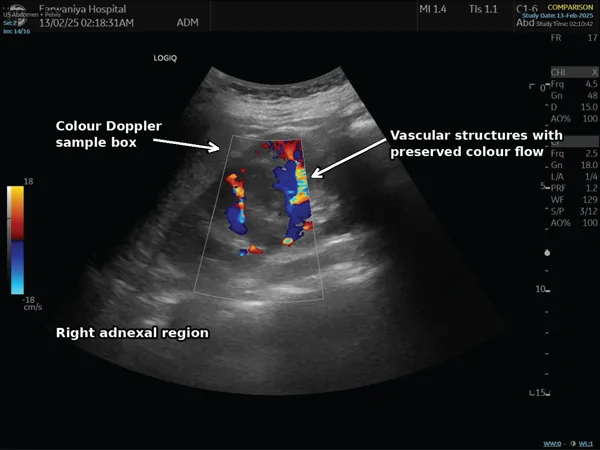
Transabdominal colour Doppler ultrasound of the right adnexal region. The colour Doppler sample box and vascular structures showing preserved colour flow are annotated. The small simple right adnexal cystic lesion of approximately 2.5–3 cm described in the contemporaneous radiology report is not resolved on this archived static image; the full sonographic findings are reported in the text. Preserved colour flow does not exclude tubal torsion because of the dual blood supply of the fallopian tube.
